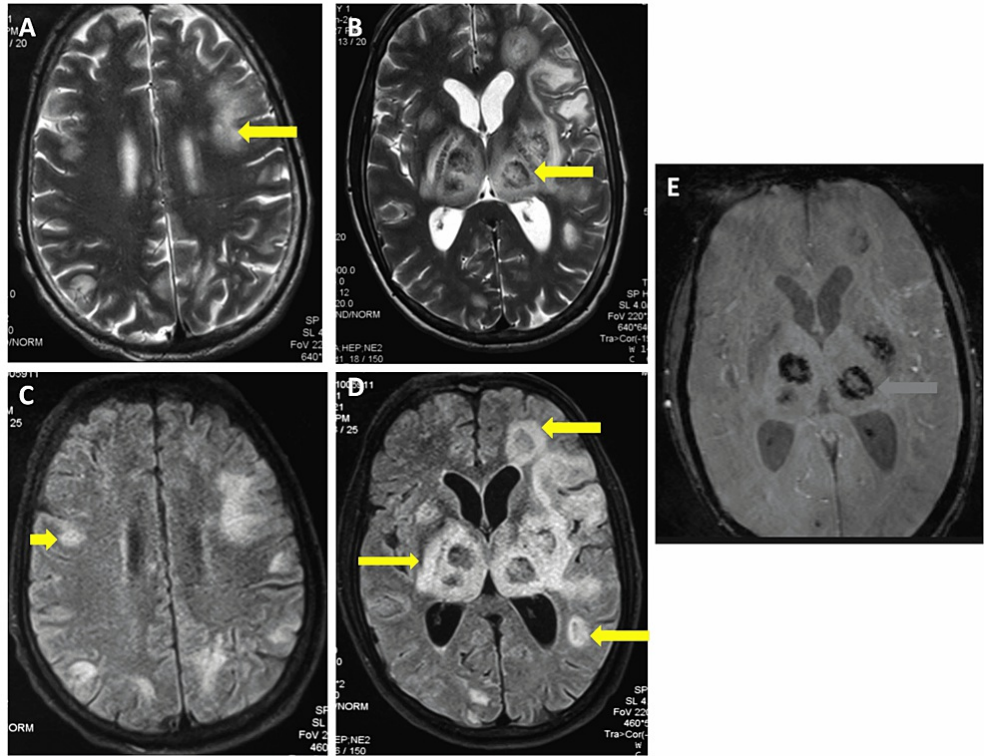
Magnetic resonance imaging of the brain (A-E) Axial T2 weighted images (A and B) and FLAIR images (C and D) show multiple hyperintense lesions (yellow arrows) in bilateral cerebral hemispheres at the corticomedullary junction, bilateral basal ganglia, and thalami, which show blooming (blue arrow) on susceptibility-weighted images (E). In an immunocompromised patient, these findings suggest toxoplasmosis.
